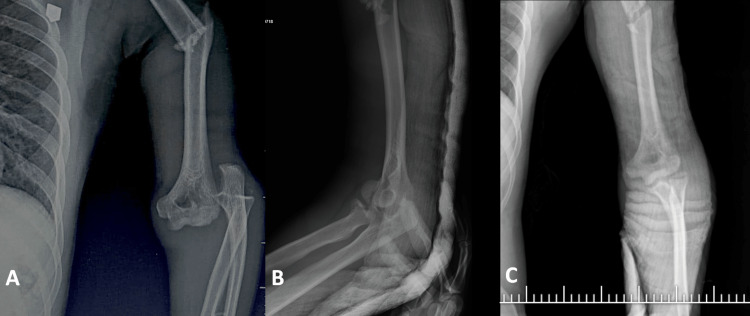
(A) X-ray of the left arm demonstrating humerus shaft fracture with an ipsilateral elbow dislocation. (B and C) Lateral X-ray view of the left arm demonstrating reduced elbow dislocation with a clear demonstration of the radial head and capitellum fractures.
